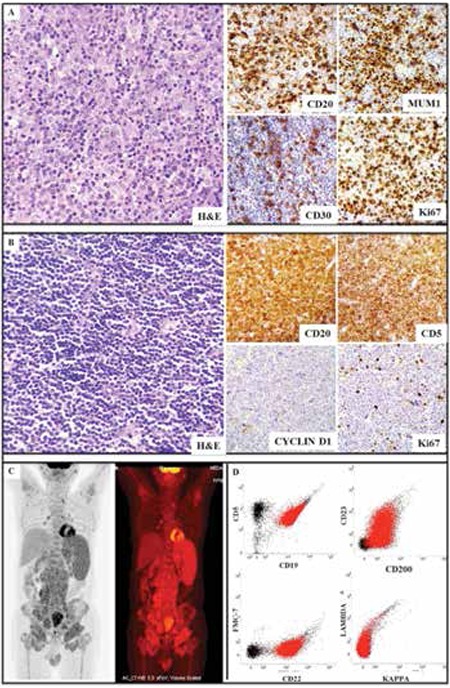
A) Lymph node biopsy showing diffuse infiltration of large atypical cells with prominent nucleoli and vesicular chromatin, which were positive for CD20, CD30, and MUM1 with a Ki67 index of 80%. B) Biopsy from paraaortic mass showing small-sized neoplastic cells with scant cytoplasm, hyperchromatic nuclei, and clumped chromatin arranged in sheets, which were positive for CD20 and CD5 and negative for cyclin D1 with a low Ki67 index. C) 18F-FDG PET-CT showing multiple cervical, supraclavicular, mediastinal, axillary, abdominal, and pelvic lymphadenopathies with gross splenomegaly. D) Immunophenotyping of peripheral blood smear showing 84% abnormal lymphoid cells, which were positive for CD19, CD5, CD23, CD22 (dim), and CD200 with lambda light chain restriction and negative for FMC7.
